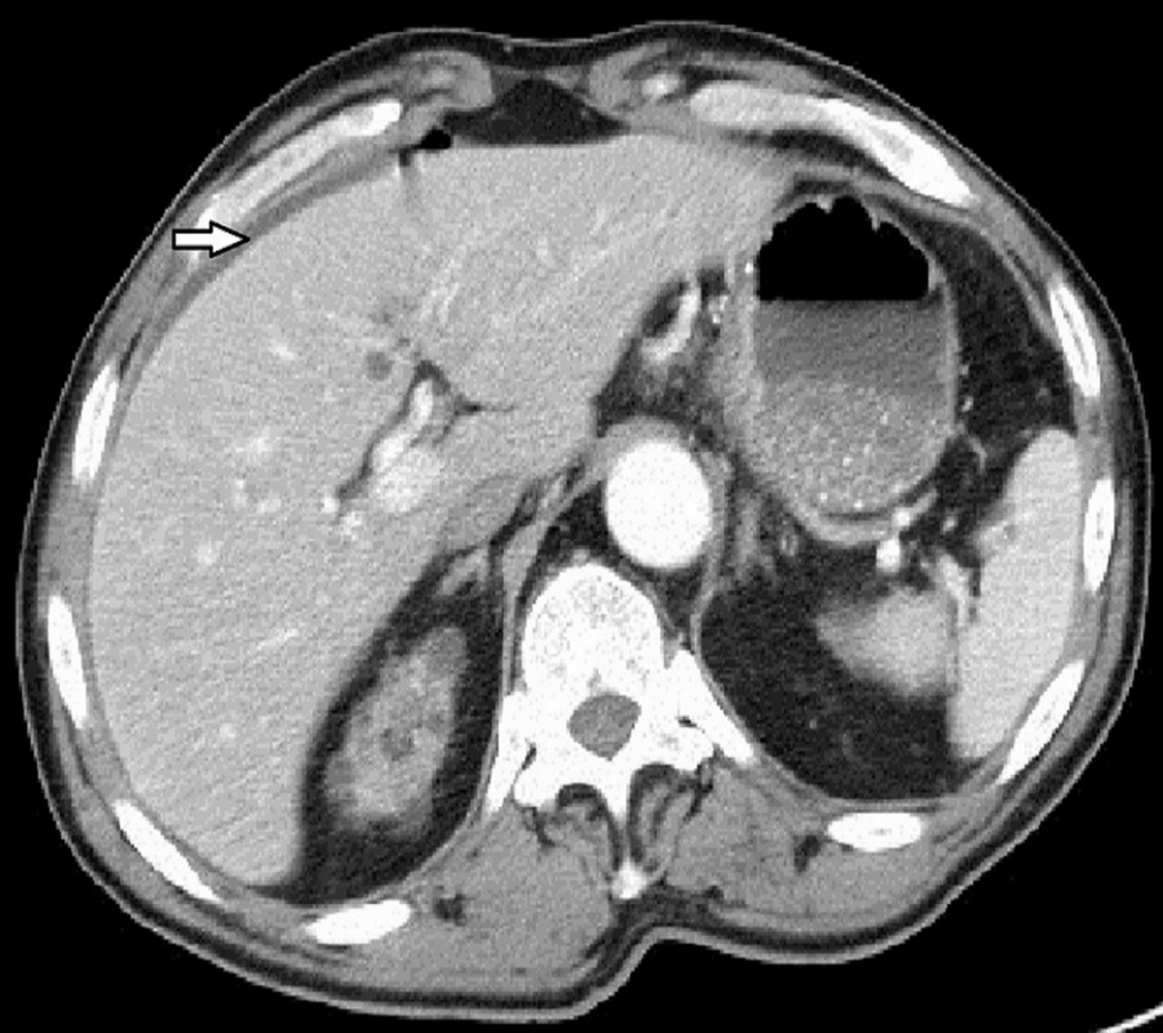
Computed tomography (CT) scan of the abdomen and pelvis showing a small amount of free fluid in the peritoneal cavity
